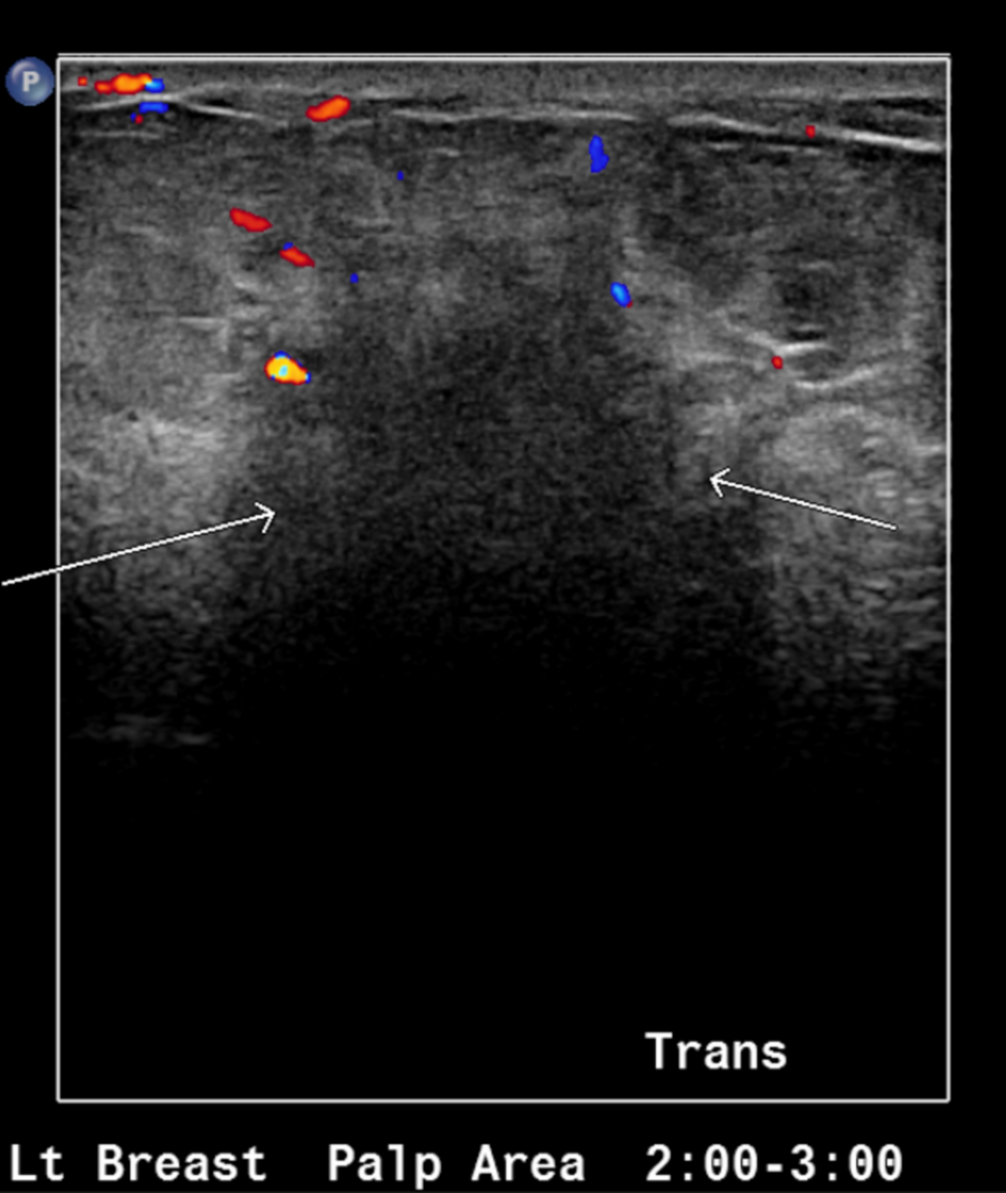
Ultrasound of the left breast showing area of abnormal echogenicity
